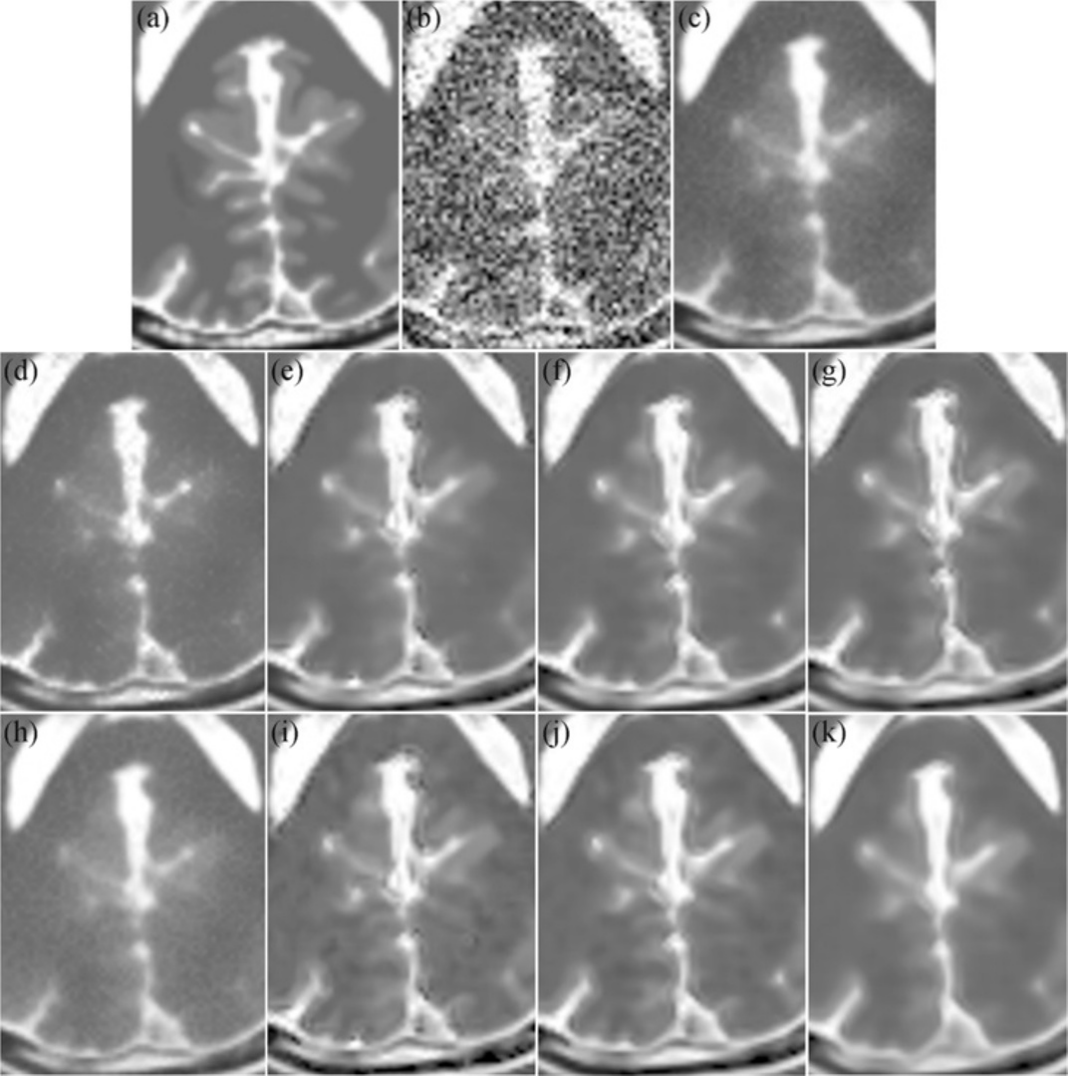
Comparison of experiment results on T2-weighted MRI. (a) original MRI, (b) noisy MRI with 17% Rician noise, (c) results of the original NLM filter, (d) results of the UNLM with squared magnitude transformation, (e) results of the PSNLM1 with median filter, (f) results of the PSNLM1 with Gaussian filter, (g) results of the PSNLM1 with anisotropic filter, (h) results of the UNLM with VST, (i) results of the PSNLM2 with median filter, (j) results of the PSNLM2 with Gaussian filter, (k) results of the PSNLM2 with anisotropic filter.
